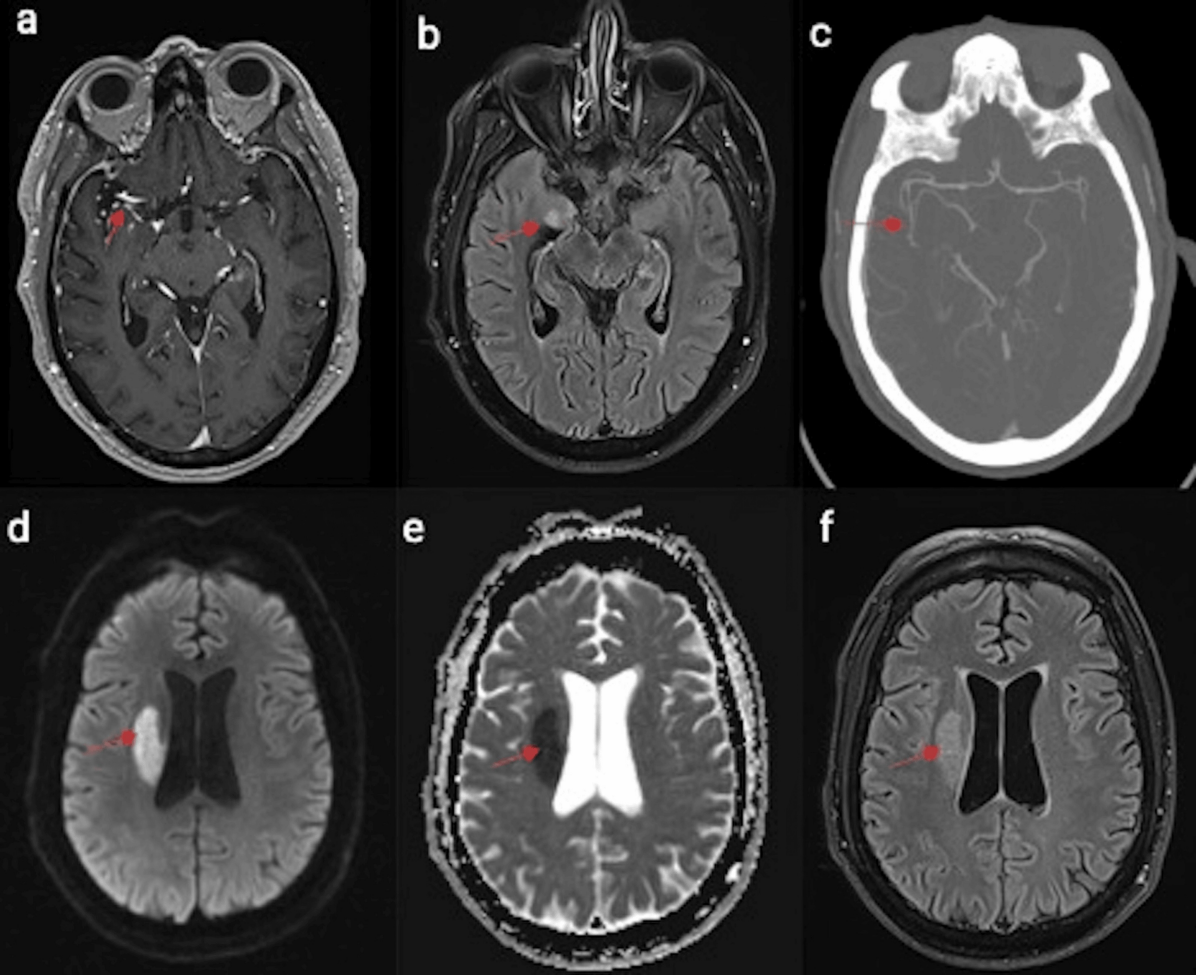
Magnetic resonance imaging (MRI) of the brain and computed tomography angiography (CTA) of the brain Diffusion-weighted imaging (DWI) hyperintensity (d: red arrow)/decreased apparent diffusion coefficient (ADC) (e: red arrow) and increased fluid-attenuated inversion recovery (FLAIR) signal (f: red arrow) in the right corona radiata, putamen, and amygdala (d, e, f, b) correspond to likely a secondary perforating infarct with foci of adjacent leptomeningeal enhancement along the lenticulostriate perforators (a: red arrow). CTA demonstrates right proximal M2 narrowing (c: red arrow).
